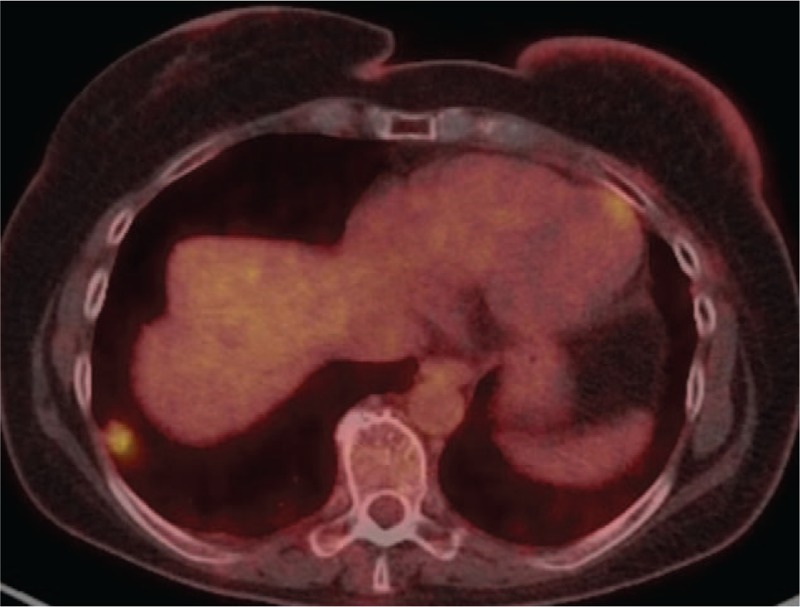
Pet/CT scan fused axial: RLL pet positive nodule.
